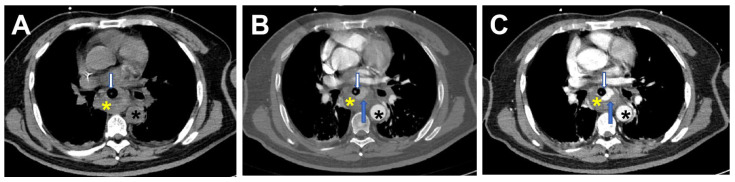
CTA examination: Non-contrast scan (A), arterial phase (B), and portal venous phase (C) images demonstrate a high-density enhancing shadow in the esophageal lumen (yellow *) on the non-contrast scan. During the arterial phase, a “crescent-shaped” higher-density shadow (blue arrow) is observed, which increases over time (C), suggesting active bleeding within the esophagus. A triple-lumen two-balloon tube is seen in the esophageal lumen (white arrow). Descending thoracic aorta (black *).
